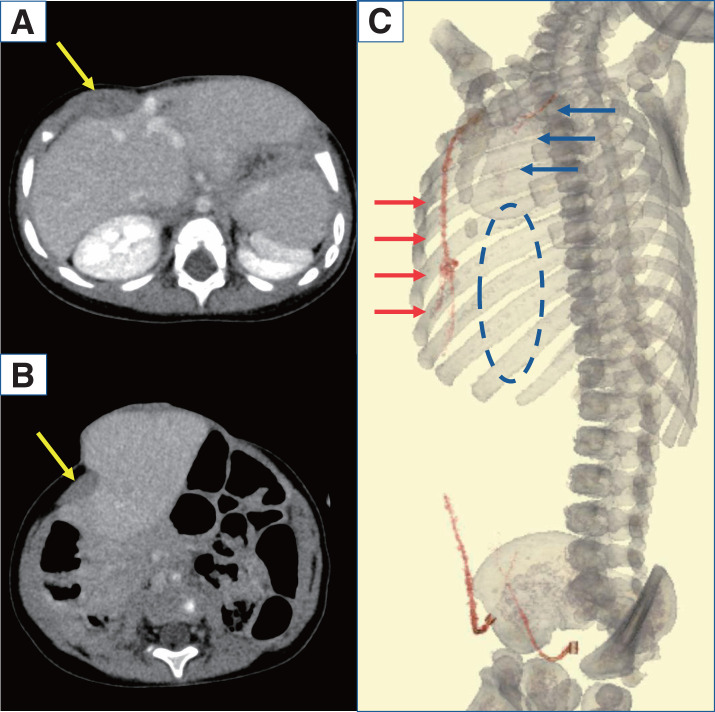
Contrast CT before the 2nd operation. Contrast CT images taken before the 2nd operation. On the axial plane, the rectus abdominis muscle can be seen on the right (arrow) but is absent on the left (A, B). On vascular reconstruction imaging, the area from the internal thoracic artery to the superior epigastric artery was able to be confirmed on the right side (red arrow). On the left side, the internal thoracic artery appeared thin (blue arrow), and the presence of the superior epigastric artery was not able to be confirmed (blue dotted circle). The inferior epigastric arteries were identifiable on both sides (C).
